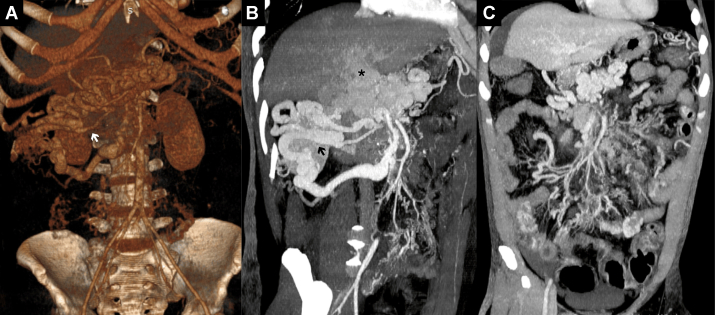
Computed tomography findings of chronic portal vein thrombosis with cavernous transformation and mesenteric arteriovenous malformation (AVM). (A) Three-dimensional reconstruction shows cavernous transformation at the hepatic hilum with dilated mesenteric veins (*arrow*). (B) Arterial-phase sagittal computed tomography demonstrates dilated mesenteric branches (*arrow*) and diffuse AVM at the hilum (*asterisk*) and mesentery. (C) Coronal venous-phase computed tomography reveals tortuous, dilated mesenteric veins consistent with venous congestion and arteriovenous fistulas.
